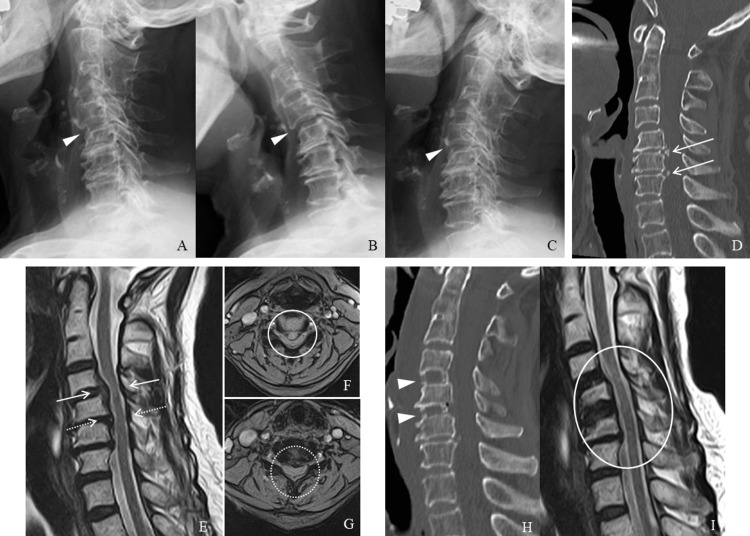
Pre- and postoperative cervical imaging examinations A-G: Preoperative cervical imaging examinations. A-C: Lateral views of the cervical spine X-ray showing instability at C4-5 (arrowhead). D: Computed tomography shows mild posterior longitudinal ligament ossification at the C4-6 levels (arrows). E: Magnetic resonance image, sagittal view, shows cervical disc herniation at C4-6 levels (arrows: C4/5, dotted arrows: C5/6). F, G: Magnetic resonance image, axial views, demonstrating high compression of the spinal cord (F: circle, C4/5, G: dotted circle, C5/6). H, I: Postoperative cervical imaging examinations showing good cage positions (arrows) and spinal decompression (circle) (H: computed tomography; I: magnetic resonance image).
